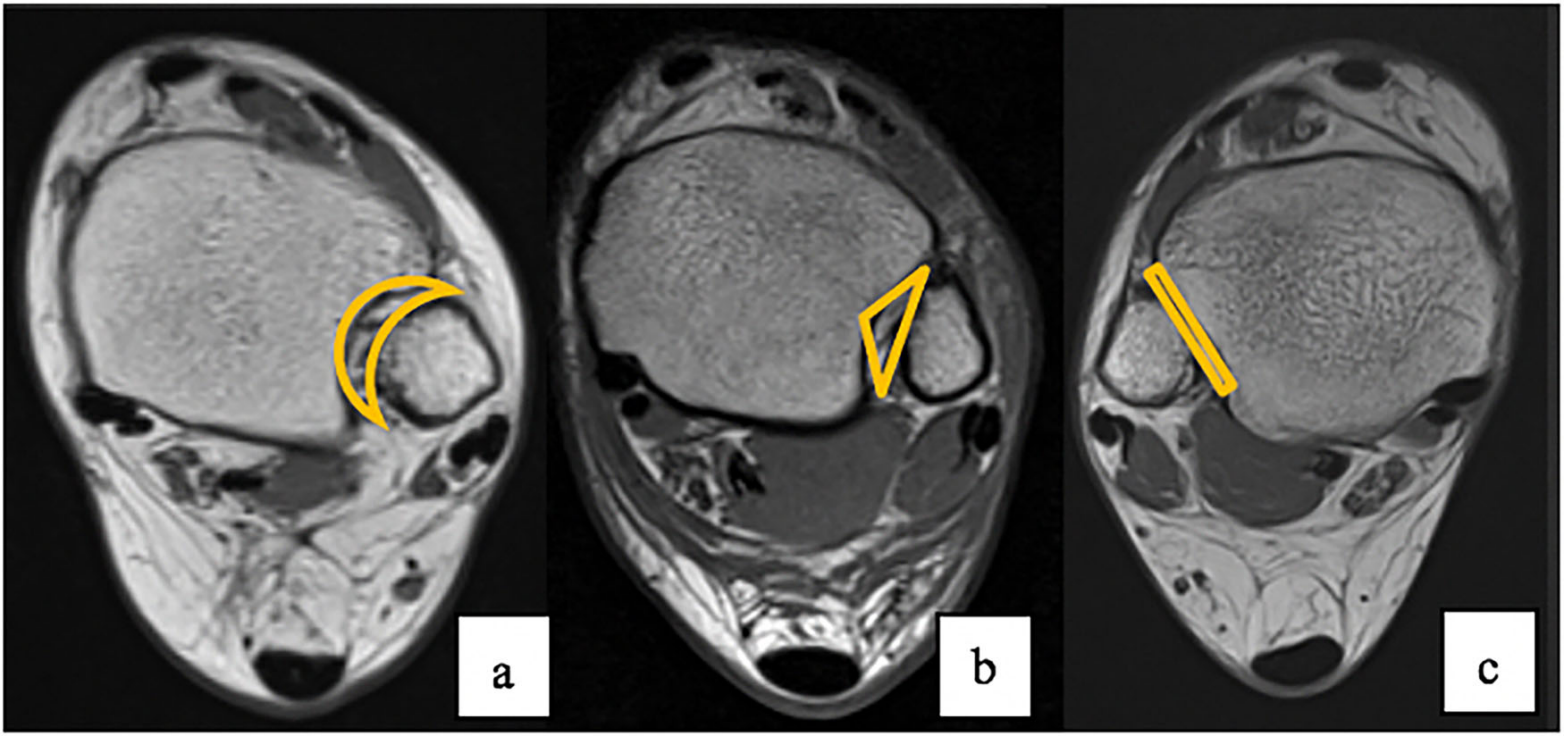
MRI T1-weighted images on the axial plane: Different morphologies of the distal tibiofibular joint anatomy. a Crescent-shaped; b Triangular-shaped; c Flat-shaped
